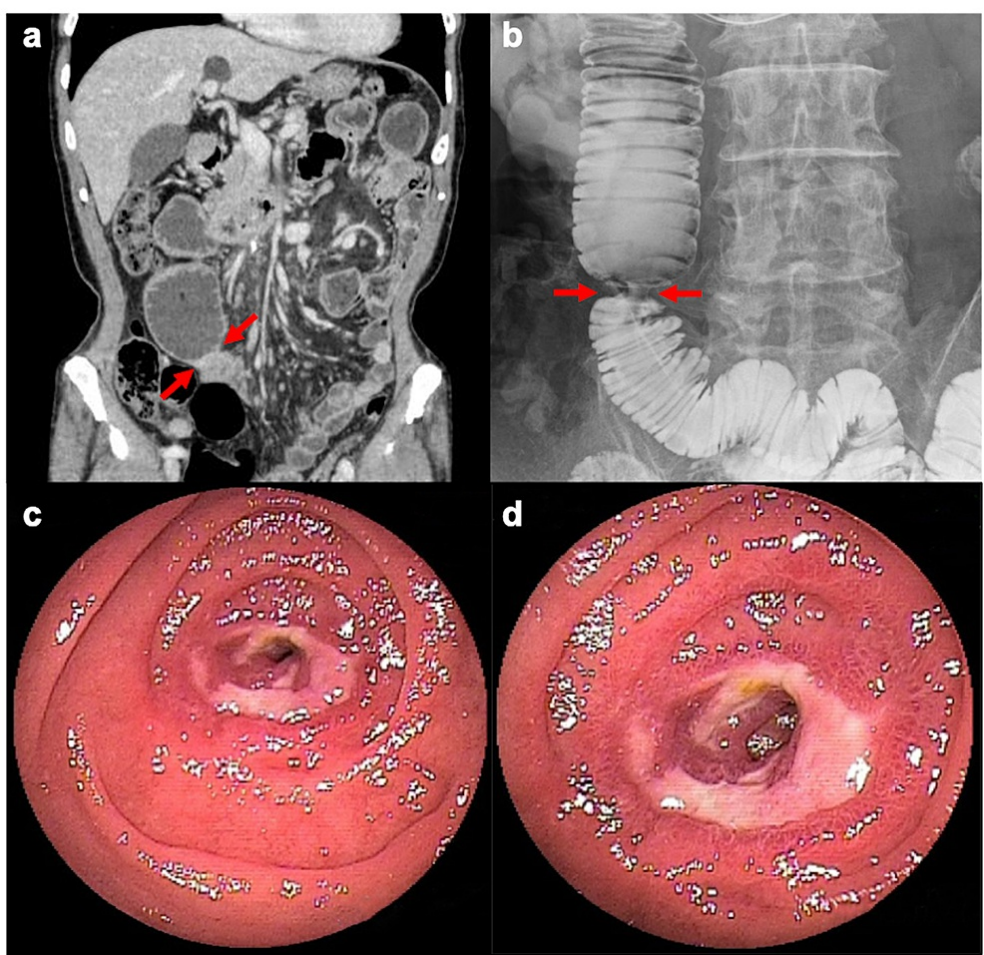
CT abdomen revealed a thickening of the small bowel wall with dilation of the proximal bowel (a, arrows). Small bowel series revealed severe jejunal stenosis (b, arrows). Antegrade double-balloon enteroscopy revealed severe stenosis with a circumferential ulcer in the jejunum (c, distant image; d, closeup image).
